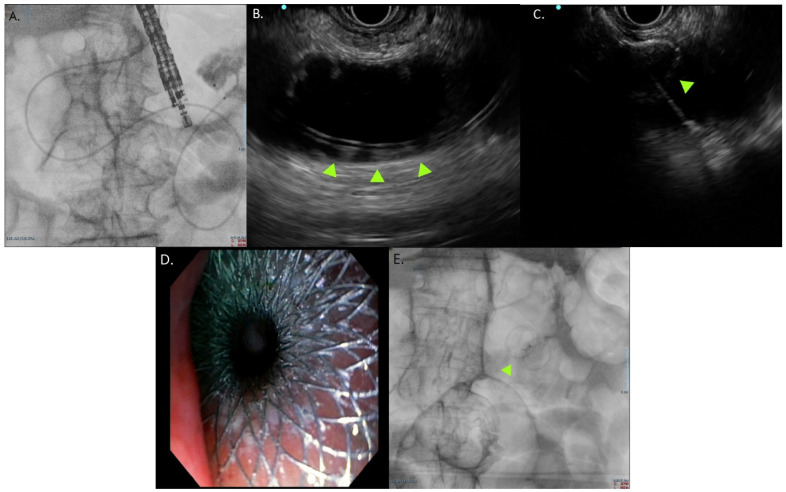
Gastroenterostomy steps. (A) Jejunal loop is distended with contrast medium and saline solution after the insertion of a nasojejunal tube. (B) Ultrasound view of the distended jejunal loop. The green arrows show the nasojejunal tube within the loop lumen. (C) Ultrasound view of the opening of the distal flange of the LAMS (green arrow) within the jejunal loop lumen. (D) Endoscopic view of the proximal flange of the LAMS in the gastric lumen. Through the stent, the jejunal mucosa can be visualized. (E) Final fluoroscopic view of the LAMS (green arrow).
